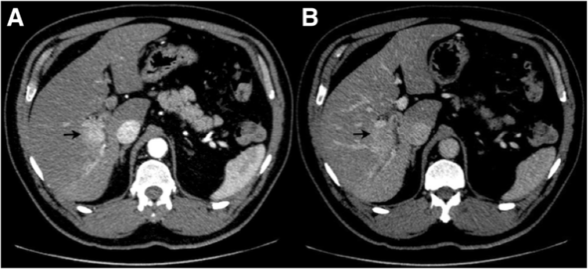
Dual phase abdominal contrast CT scan. Tumour at the bifurcation of the right anterior and posterior portal vein (*arrow*). Enhancement of lesion during arterial phase (a), with mild contrast washout in portal venous phase (b). Liver shows background steatosis
